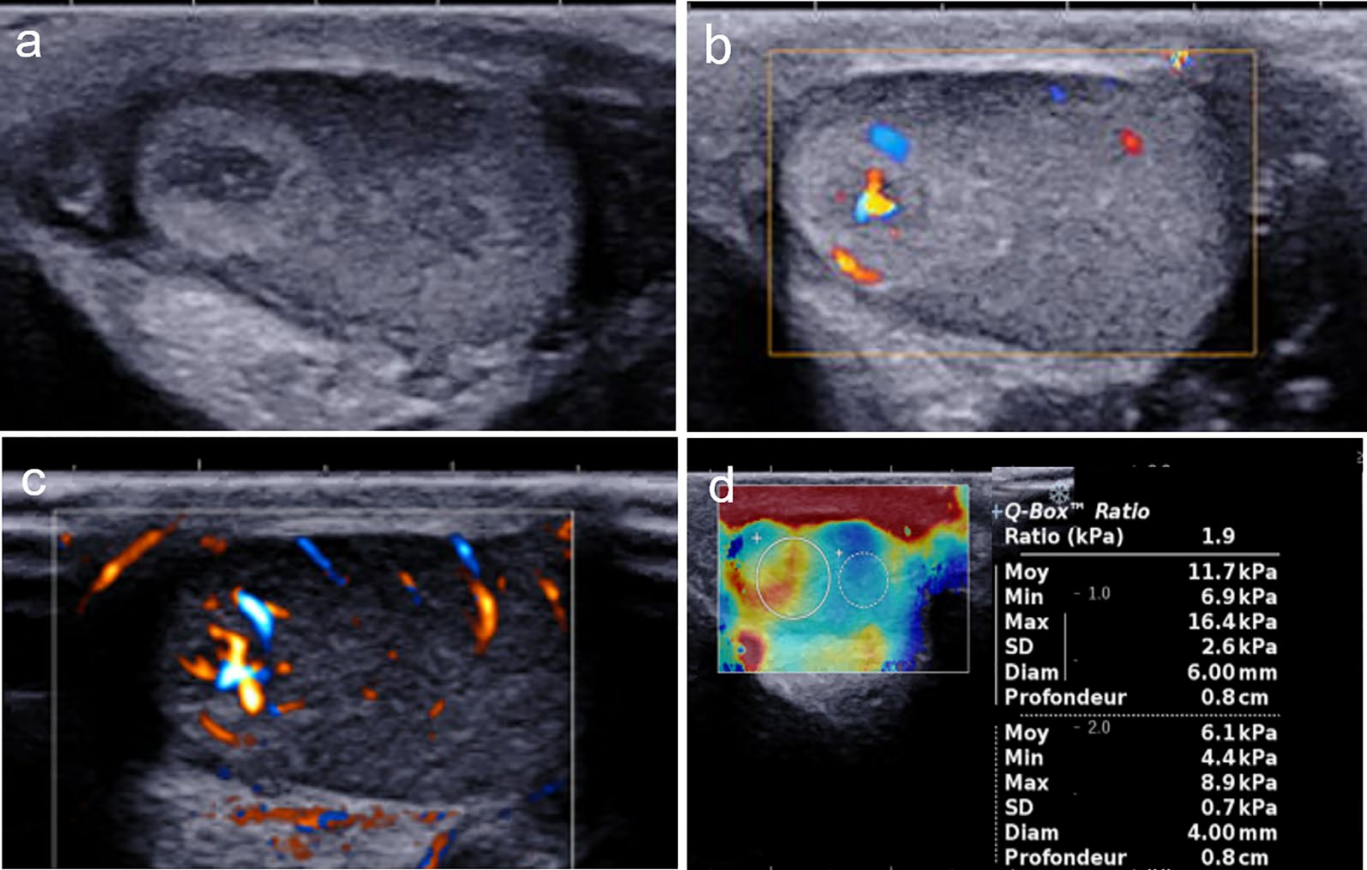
Multiparametric ultrasound. Ultrasonography of the left testicle: the tumor appeared as a hypoechoic mass surrounded by a hyperechoic rim in B-mode ultrasound (a), presenting predominant central vascularization in color Doppler (b) and ultrasensitive Doppler (c). Using shear-wave elastography, the tumor exhibited a moderate high stiffness (11.7 kPa) compared the adjacent parenchyma (6.1 kPa). Or right testicle (5.4 kPa, not shown)
